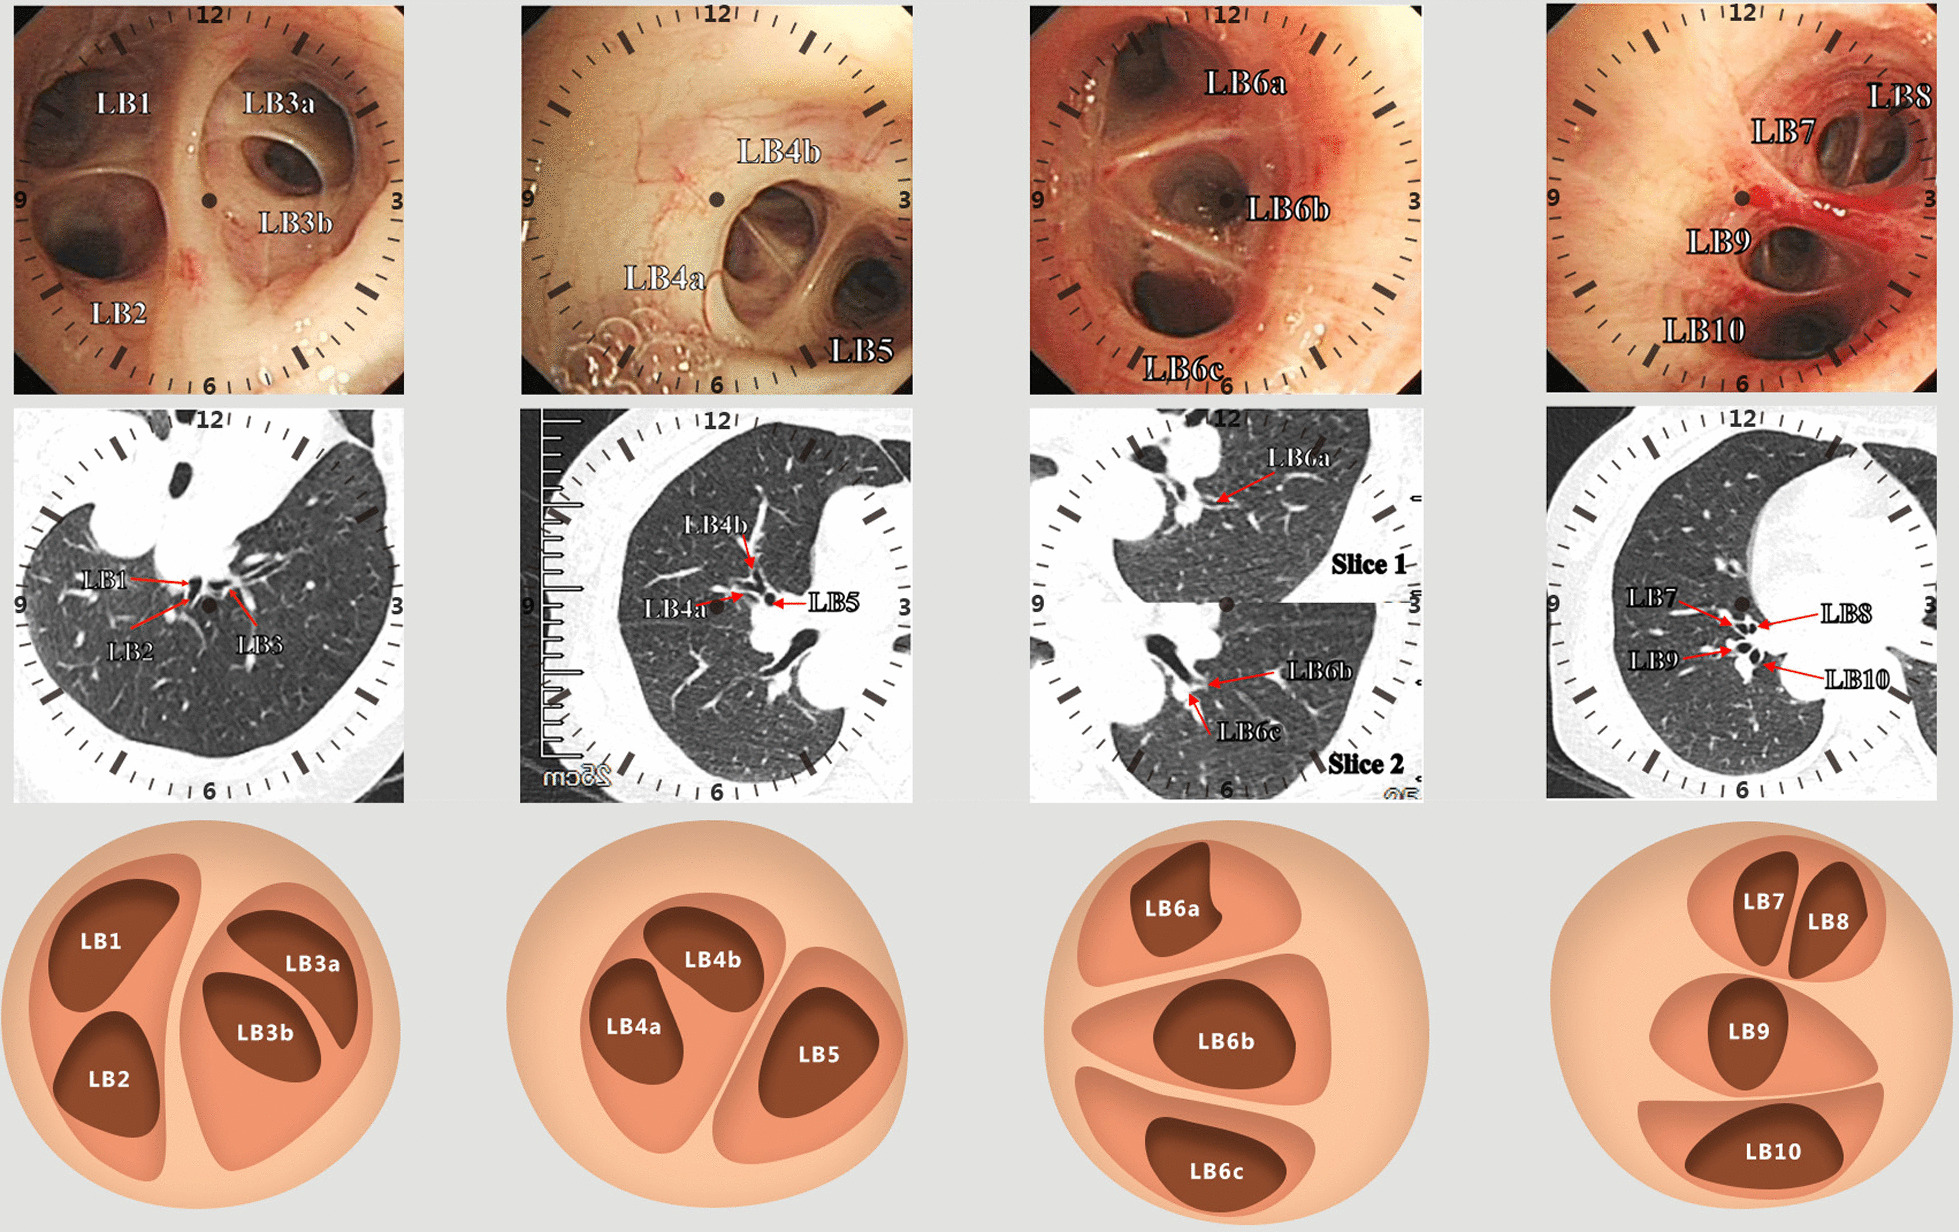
Bronchoscopy, CT image and navigation mapping of the right lung
